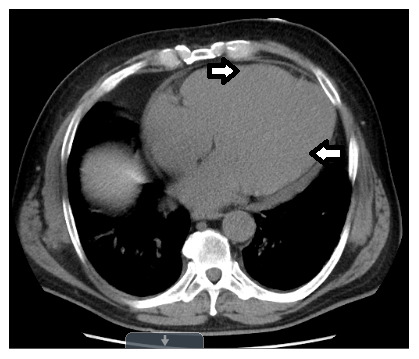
CT of chest without contrast showing left ventricular and right ventricular enlargement.
